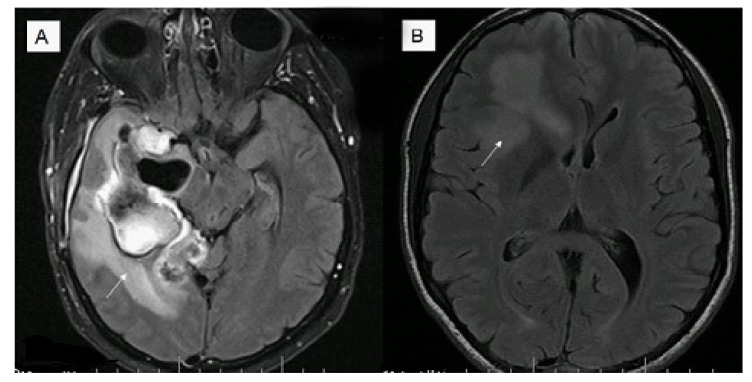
Magnetic resonance fluid attenuation inversion recovery (FLAIR) T2 imaging (A) of a 39 year old male, O(6)-methlyguanine-DNA-methyltransferase (MGMT) methylated glioblastoma (GBM) with increased edema (survival eight months post diagnosis); (B) 32 year old male, MGMT methylated GBM with less edema (alive seven years post diagnosis).
